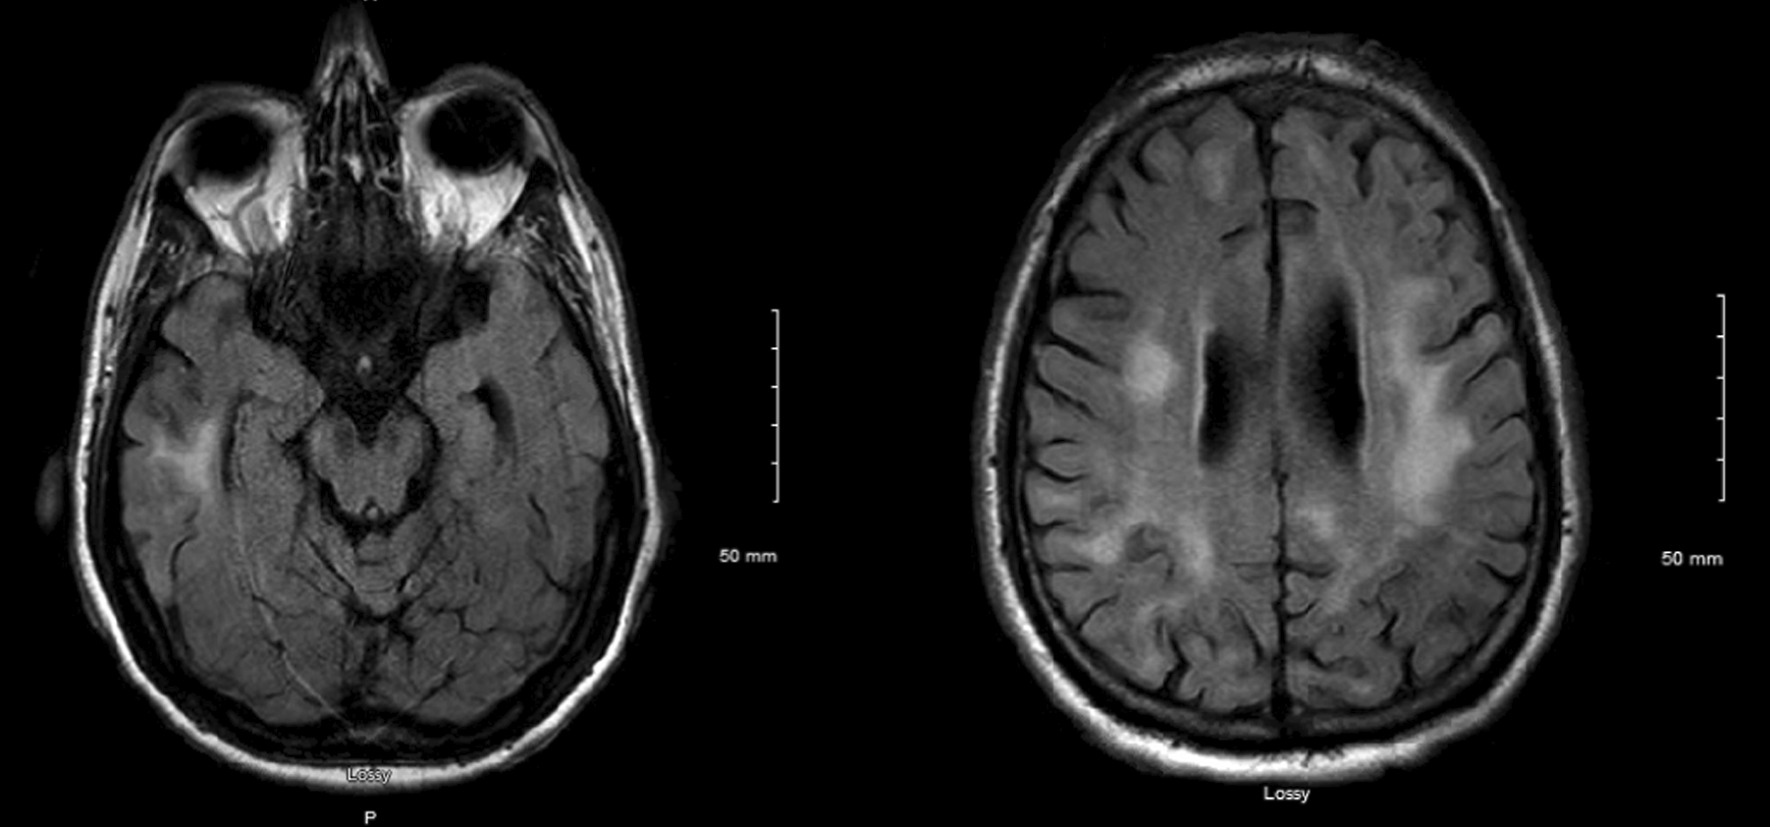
FLAIR signal brain MRI showing extensive subcortical white matter hyperintense lesions
